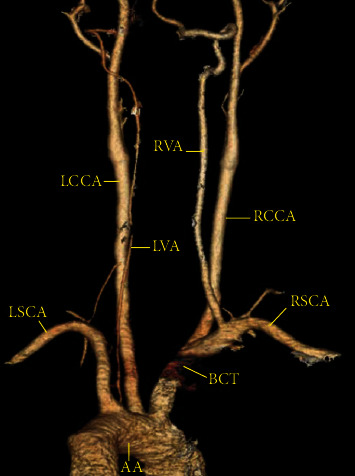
Three-dimensional reconstructed computed tomography angiography shows the carotid, subclavian, and vertebral arteries. A hypoplastic left vertebral artery originates from the aortic arch and is smaller than the right vertebral artery along its entire course. All mentioned vascular structures show normal caliber and course, smooth intima, and no narrowing or obliteration. AA = aortic arch; BCT = brachiocephalic trunk; LCCA = left common carotid artery; LSCA = left subclavian artery; RCCA = right common carotid artery; RSCA = right subclavian artery; LVA = left vertebral artery; RVA = right vertebral artery.
